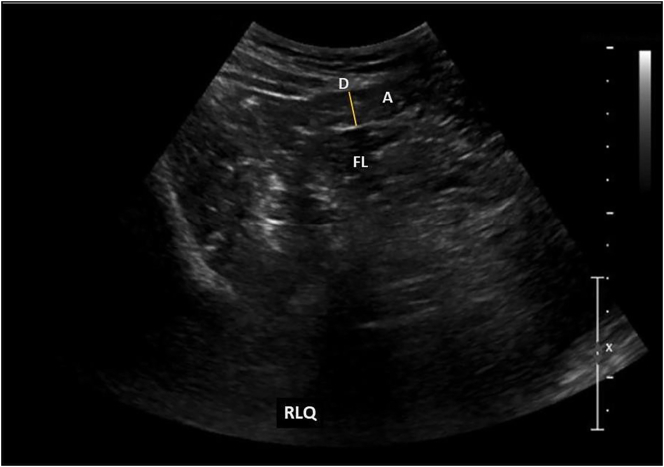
Abdominal ultrasound. RLQ: right lower quadrant. A: appendix. D: 6 mm outer diameter appendix. FL: fluid collection.
